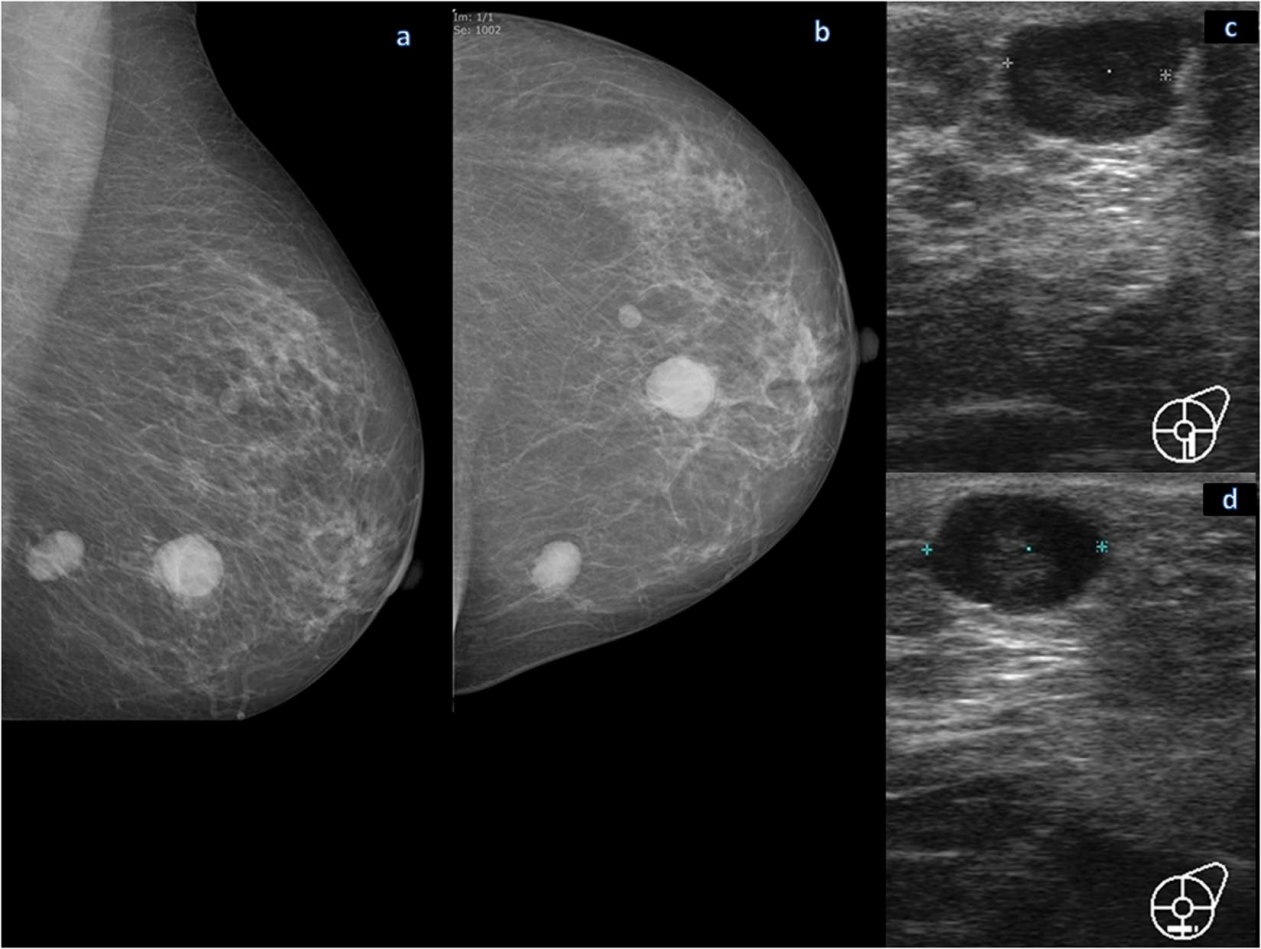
Mammographic and ultrasound findings of a leukemic relapse (acute lymphatic leukemia) affecting the left breast in a 34-year old woman. Mediolateral oblique (a) and craniocaudal (b) mammogram of the left breast shows three oval or round high-density masses with circumscribed margins. No associated skin thickening or ipsilateral axillary lymphadenopathy is present. The ultrasound findings in vertical (c) and horizontal (d) imaging planes demonstrated a circumscribed, oval mass with a complex cystic and solid echo pattern with posterior acoustic enhancement, located in the 6 o’clock position of the left breast.
